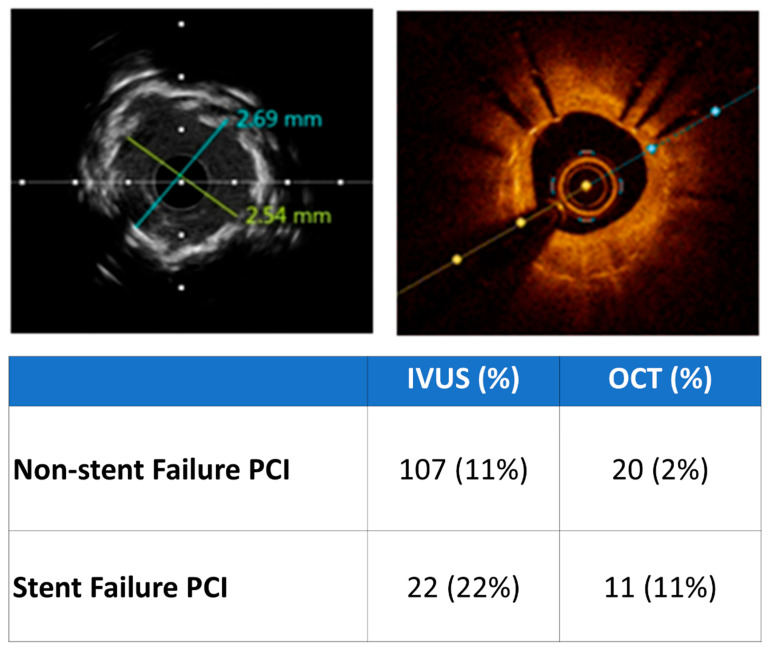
Intravascular coronary imaging penetration according the indication for percutaneous coronary intervention. IVUS, intravascular ultrasound; OCT, optical coherence tomography.
